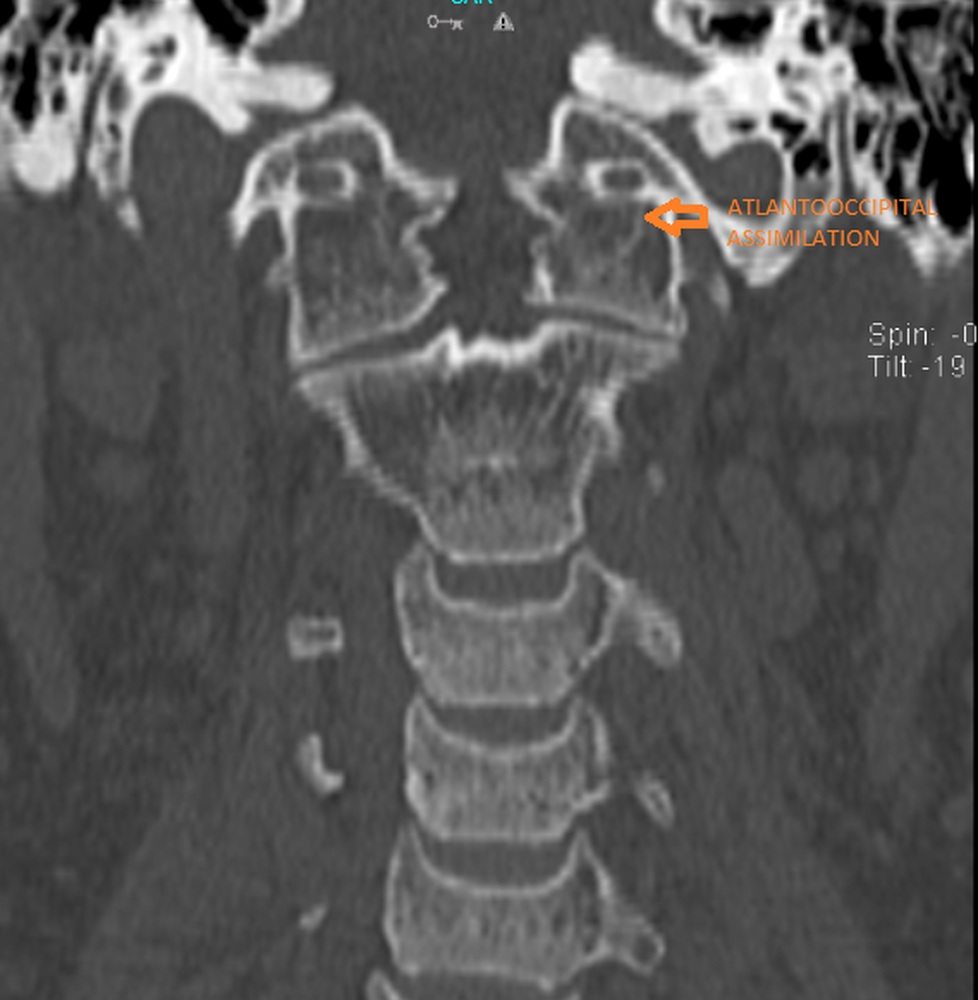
Coronal computed tomogram scan showing complete atlantooccipital assimilation (arrow)
